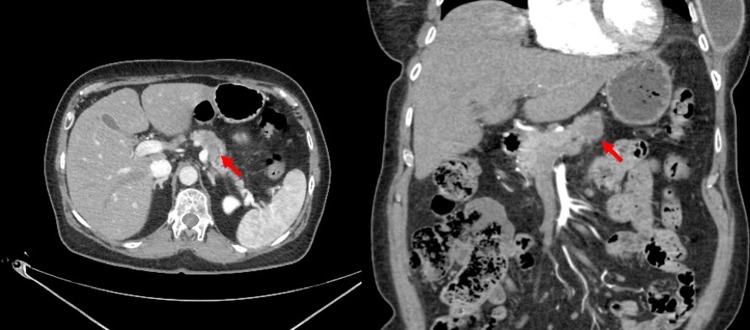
Relevant imaging for case 1: select CT imaging demonstrating a hypoattenuating and hypoenhancing lesion in the body of the pancreas (*red arrow*). CT, computed tomography.
